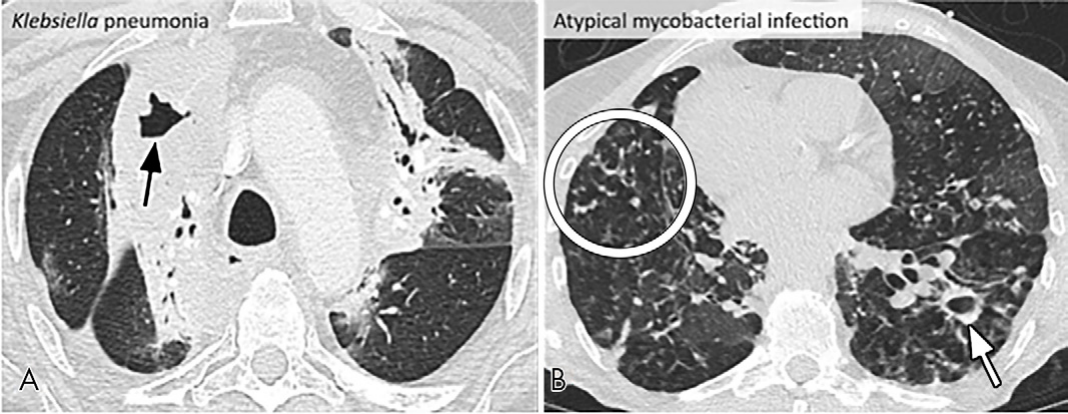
Atypical CT imaging features for COVID-19. Axial images of the lungs of two patients showing cavitation (arrow) in *Klebsiella* pneumonia (*A*) and tree-and-bud opacities (circle) and a cavity (arrow) in nontuberculous mycobacterial infection (*B*). Routine screening CT for diagnosis or exclusion of COVID-19 is currently not recommended by most professional organizations or the U.S. Centers for Disease Control and Prevention.
